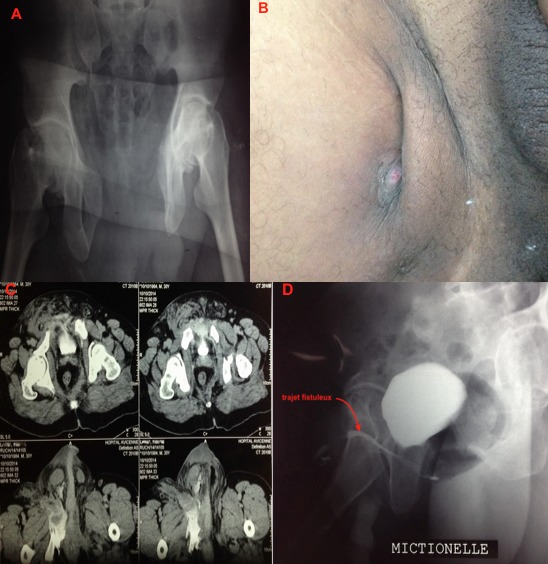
(A) radiographie du bassin montrant une importante disjonction symphysaire; (B) orifice fistuleux sur la face interne de la cuisse droite; (C) coupes tomodensitométriques pelviennes au temps tardif avec extravasation du produit de contraste à travers une plaie vésicale; (D) urétrocystographie au temps mictionnel objectivant un trajet fistuleux vesicocutane
